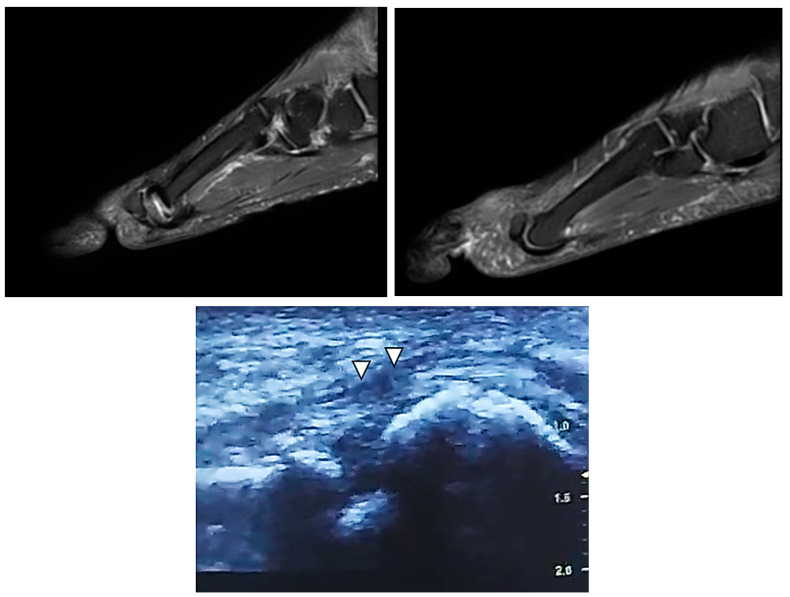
Diagnostic images corresponding to case 2. Second T2-weighted MTPJ MRI sequence with evidence of partial PP tearing (left). Normal MRI image corresponding to the healthy third MTPJ of the same foot (right). Longitudinal ultrasound image showing the full-thickness PP tear (arrows) visualized as a focal hypoechoic defect that extends through the distal substance from the articular to the plantar surface (bottom).
